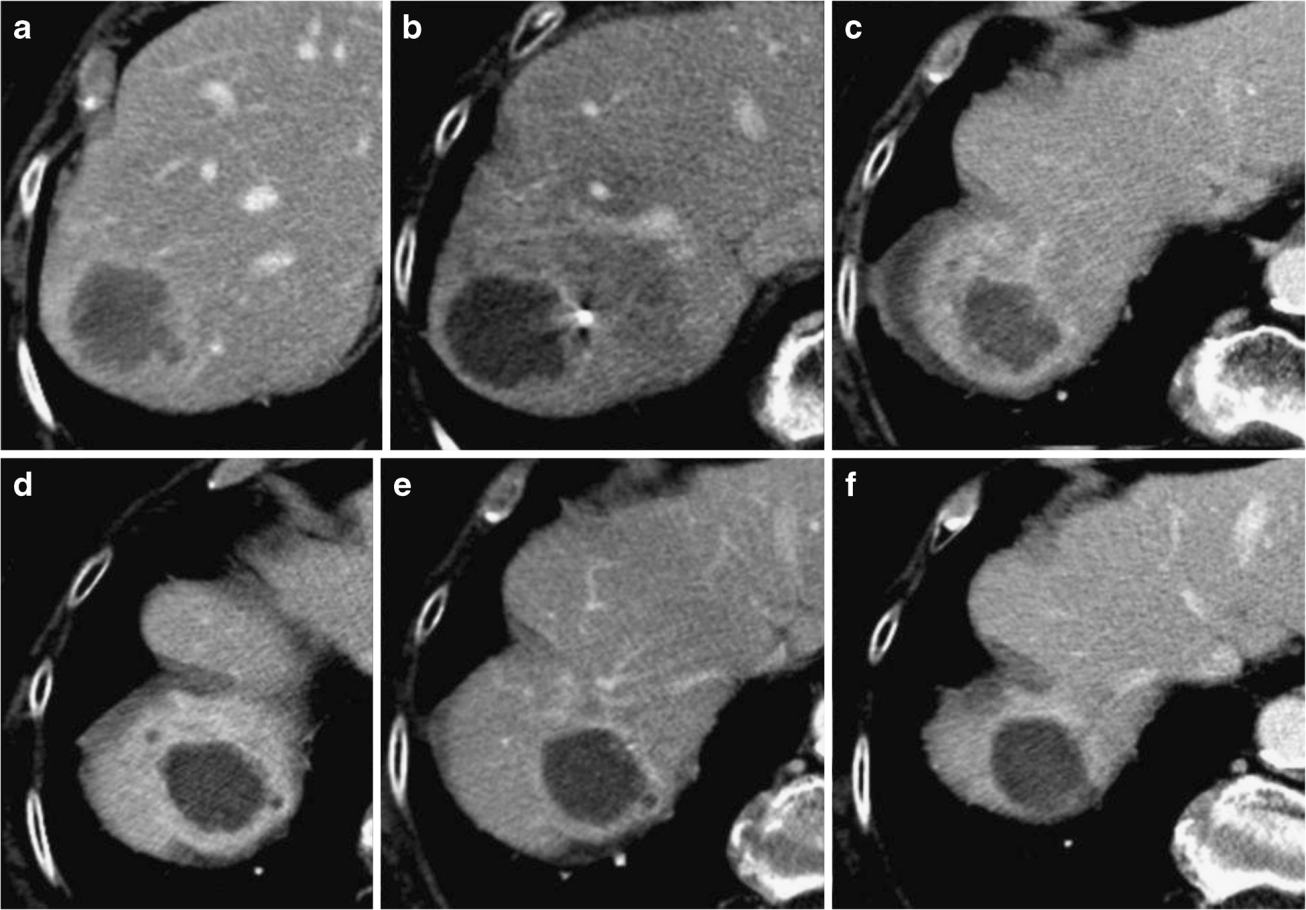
Complete response after SBRT for a liver metastasis over successive follow-ups. Before treatment (a); 2 months later (b), we observe changes of the initial phase: target-shaped lesion surrounded by a peripheral enhancement and located in a hypodense area with well delineated demarcation between irradiated and normal liver; CT slices performed at portal phase at 5 months (c); 9 months (d); 12 months (e); and 24 months later (f) show that the center is better circumscribed by a thin rim enhancement, the hypodense area progressively shrank and became hyperdense.
